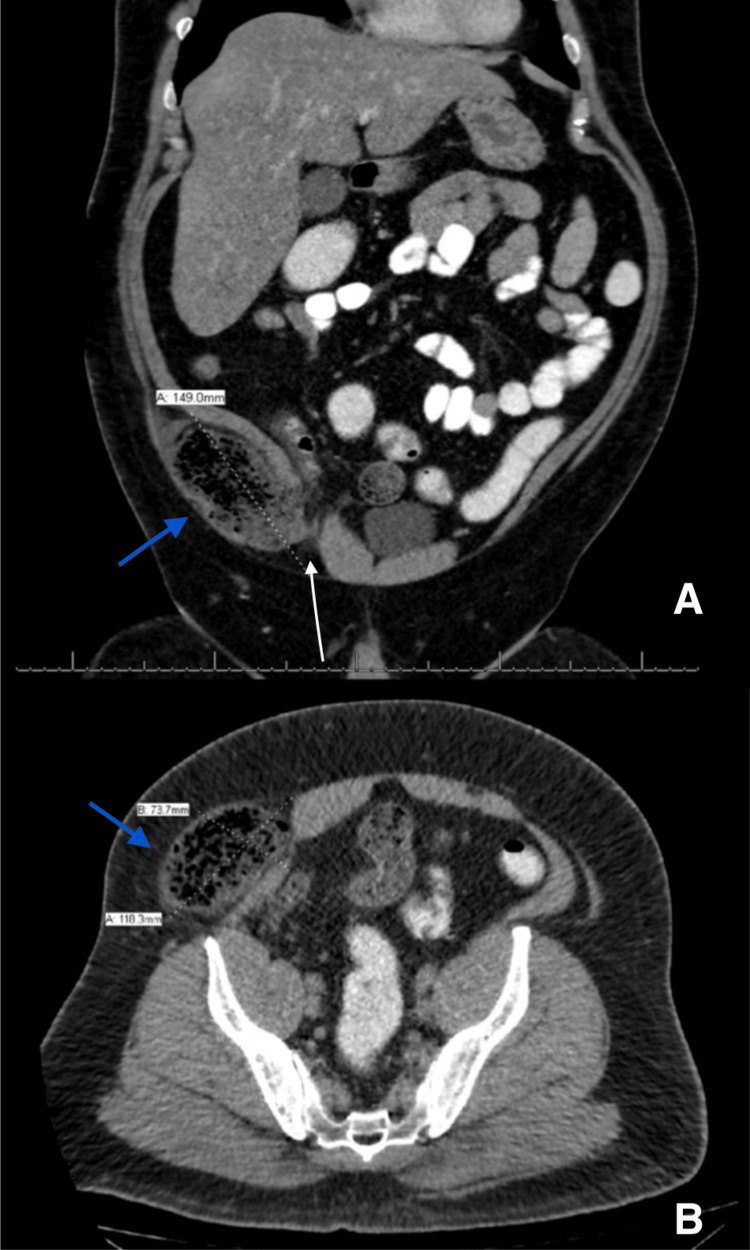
CT of the abdomen A: Coronal cut of the abdominal CT scan showing the incarcerated wall of the cecum, ileum, and appendix (blue arrow points to the herniated bowel) in relation to the inguinal region(white arrow points to the abdominal wall defect); B: Axial cut with similar findings
